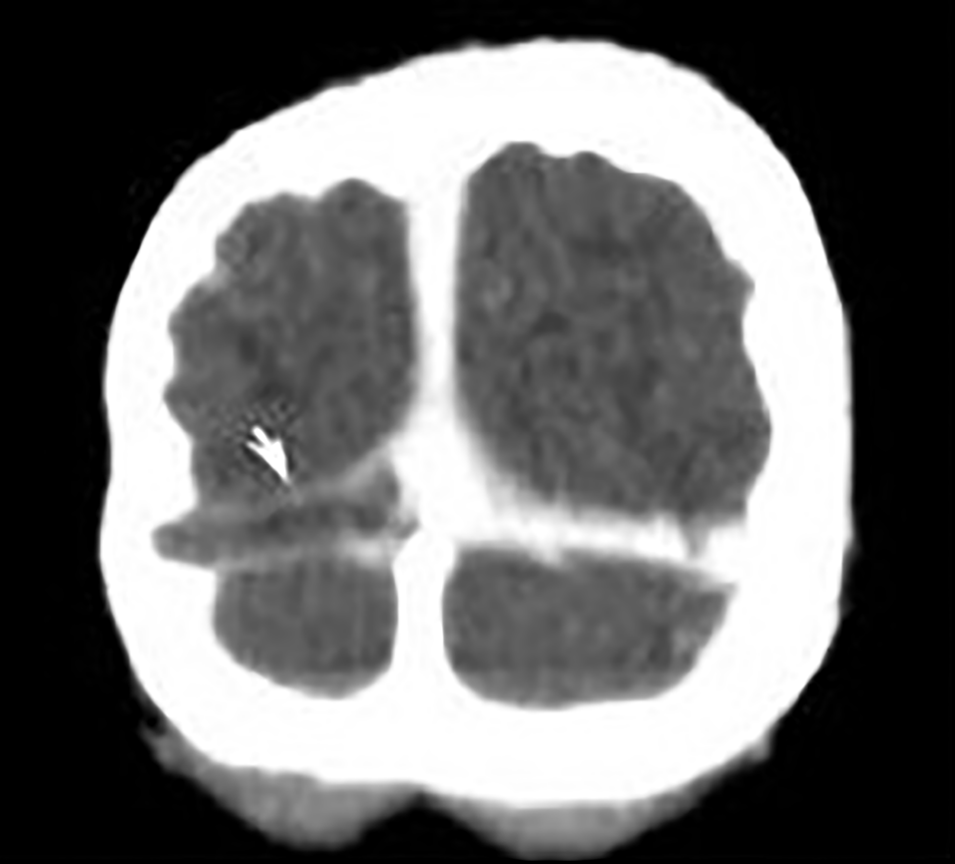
Baseline head CT scan (Electronic Patient Chart).
